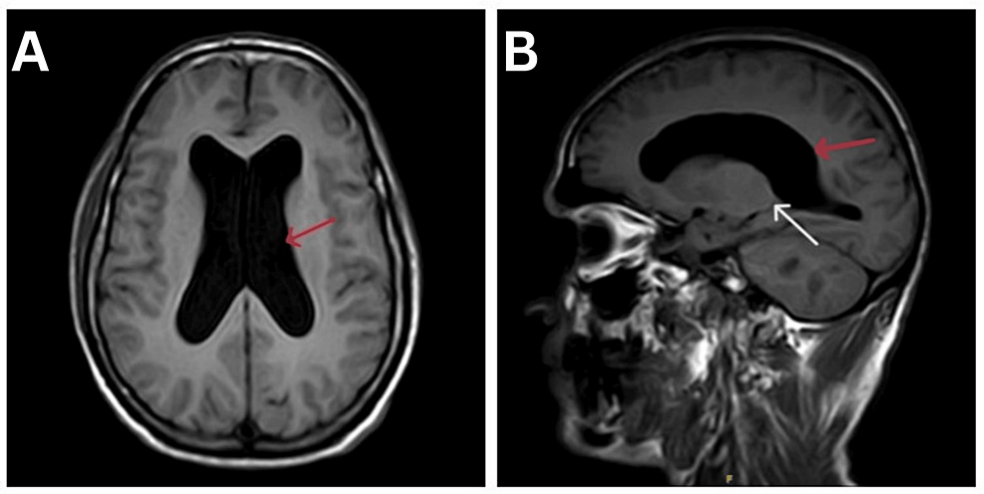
The T1-weighted sequence of the patient's brain MRI (A) Axial section and (B) sagittal section, the red arrows suggesting ventriculomegaly and the white arrow showing aqueductal stenosis MRI: magnetic resonance imaging
